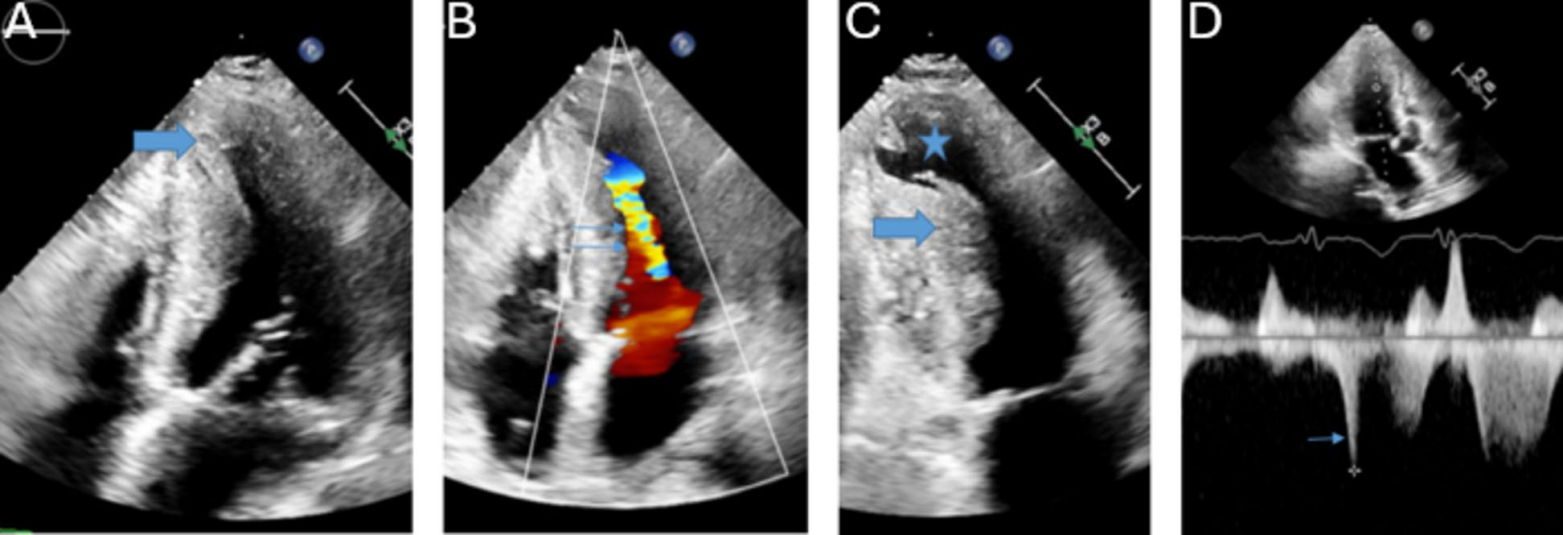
This is a patient with HCM. The thick arrows demonstrate apical (A) and mid ventricular (C) hypertrophy. The *star* denotes an apical aneurysm (C). Color Doppler (*double thin arrows*) and spectral Doppler (*single thin arrow*) demonstrate flow acceleration and an intracavitary gradient, respectively (B & D). A 36 mmHg fixed mid-cavitary obstruction was present
